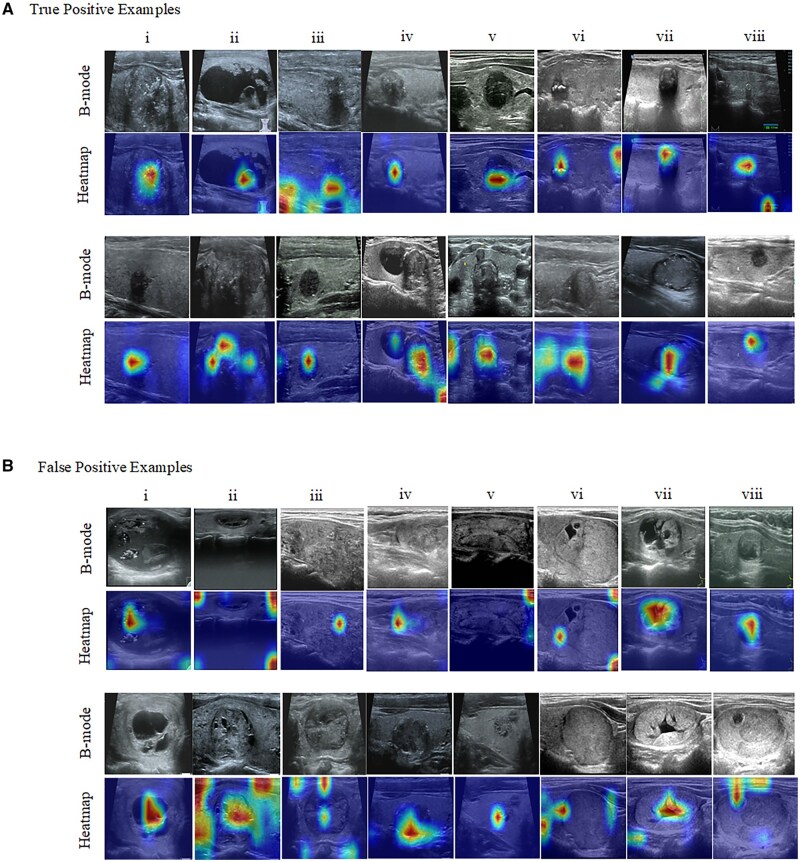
Visualization of the thyroid lesions attention heatmaps in B-mode ultrasound (US) images from the Southwest (SW) cohort. These heatmaps are generated using the deep convolution in MMOE. A) Heatmaps of true positive thyroid lesions, confirmed as malignant by both MDT-TC and pathology; B) heatmaps of false positive thyroid lesions, in which MDT-TC predicted malignant, while pathological results were benign.
